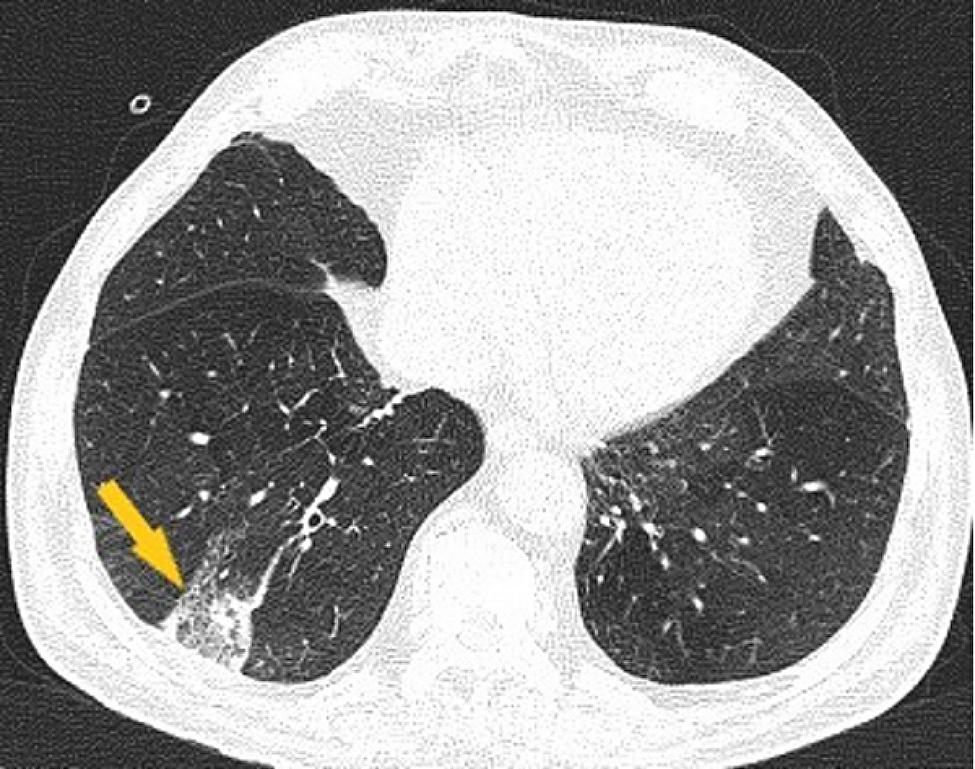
Chest CT examination, arterial time: right lower lobe with subpleural consolidation suggestive of pulmonary infarction (yellow arrow)
